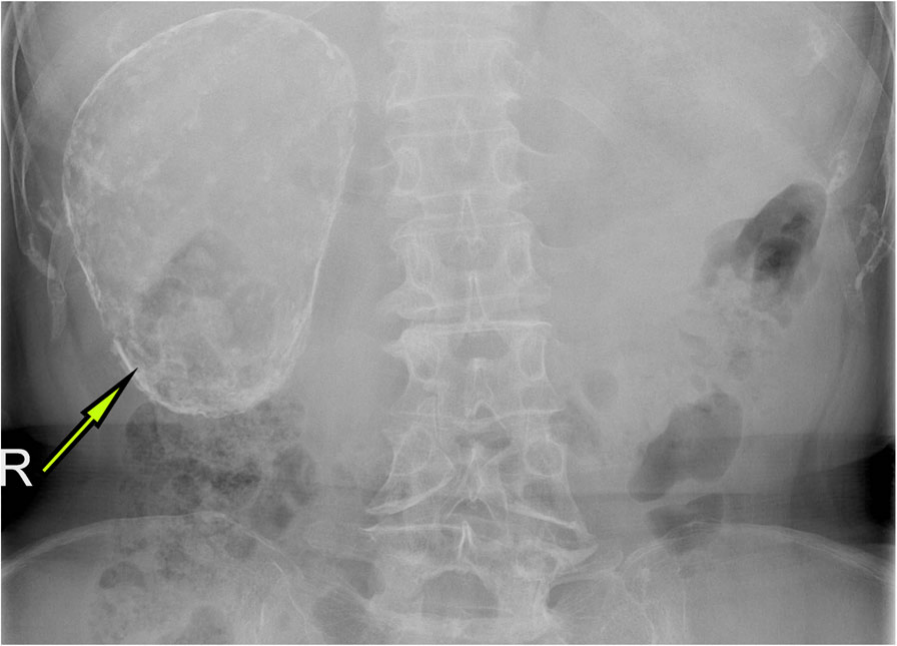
Plain X-ray image of KUB showing the high-density shadow of the right kidney, approximately 10.2 cm * 7.5 cm in size
